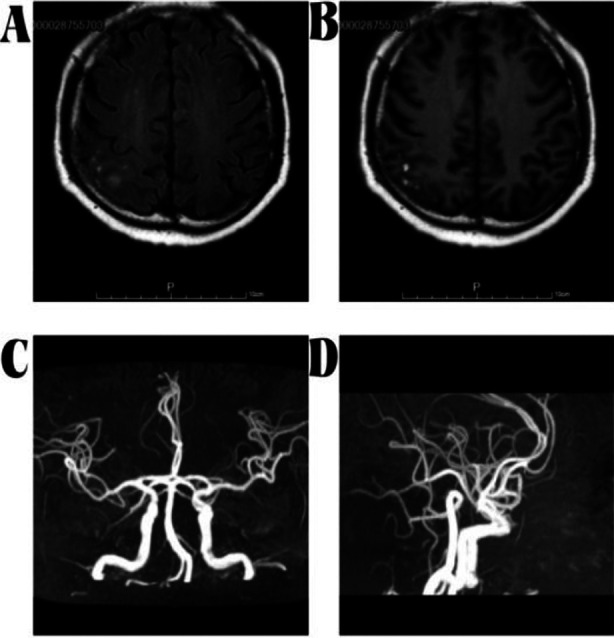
Magnetic resonance imaging of brain showed a short T1 signal shadow in the upper right sulcus of (A, B). Magnetic resonance angiography of brain showed no obvious abnormalities (C, D).
